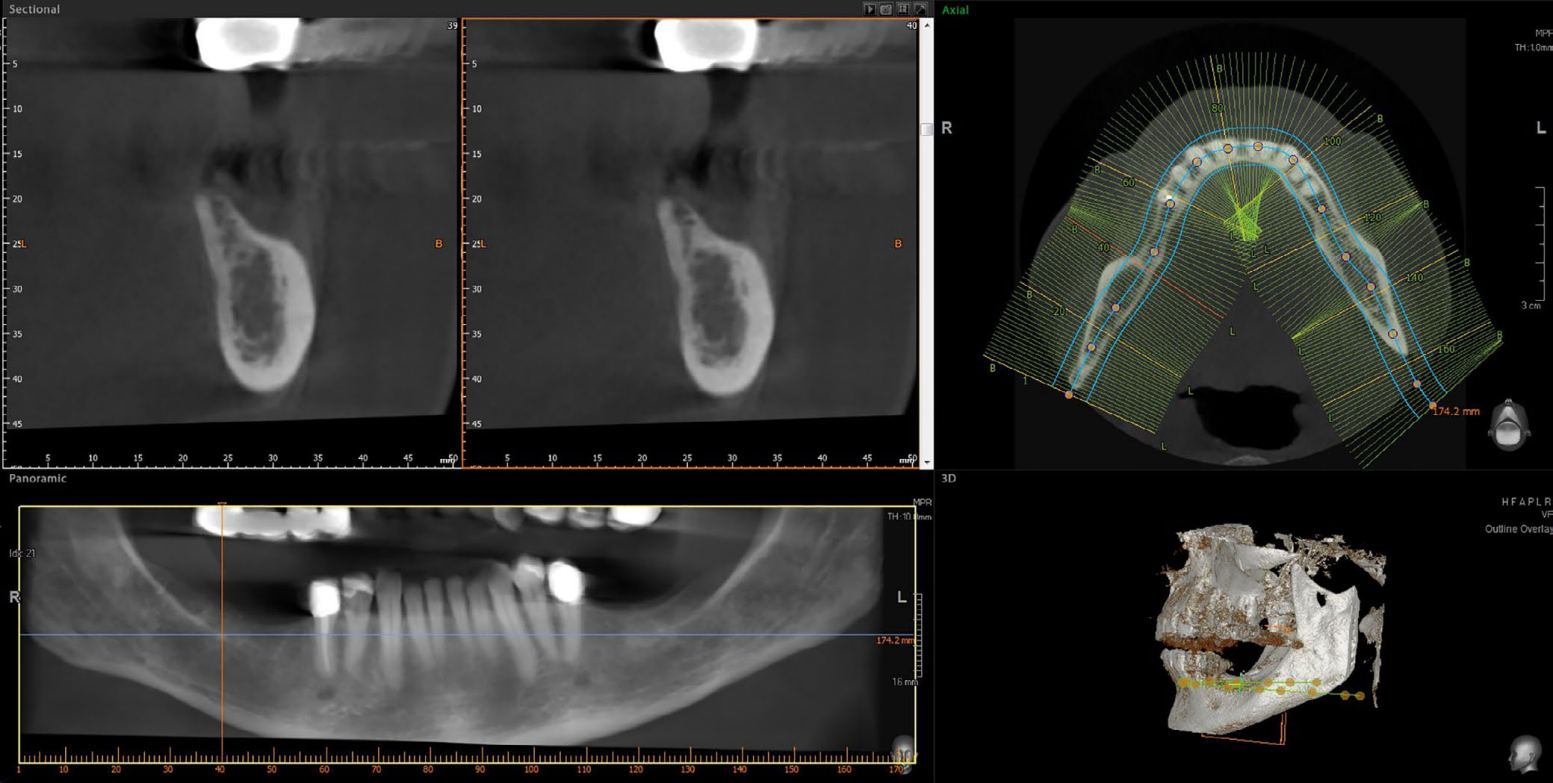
Preoperative CBCT: vertical bone defects in the third and fourth quadrant
